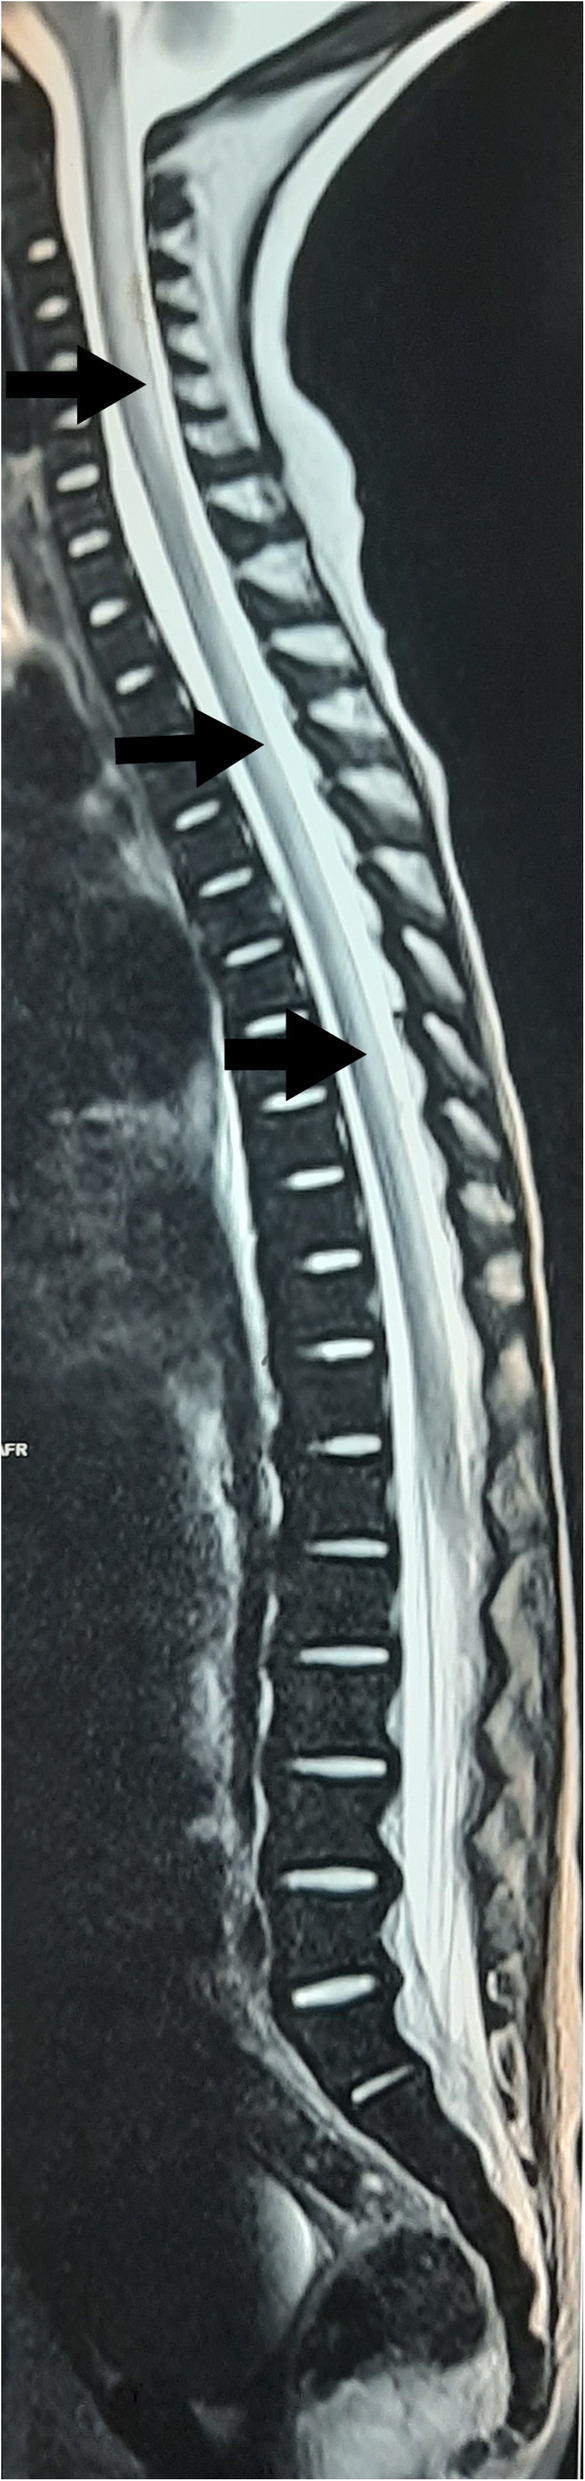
Sagittal T2-weighted MRI image showing increased signal intensity (arrows) in posterior columns of the spinal cord extending from cervico-medullary junction to the upper border of the T12 vertebra
